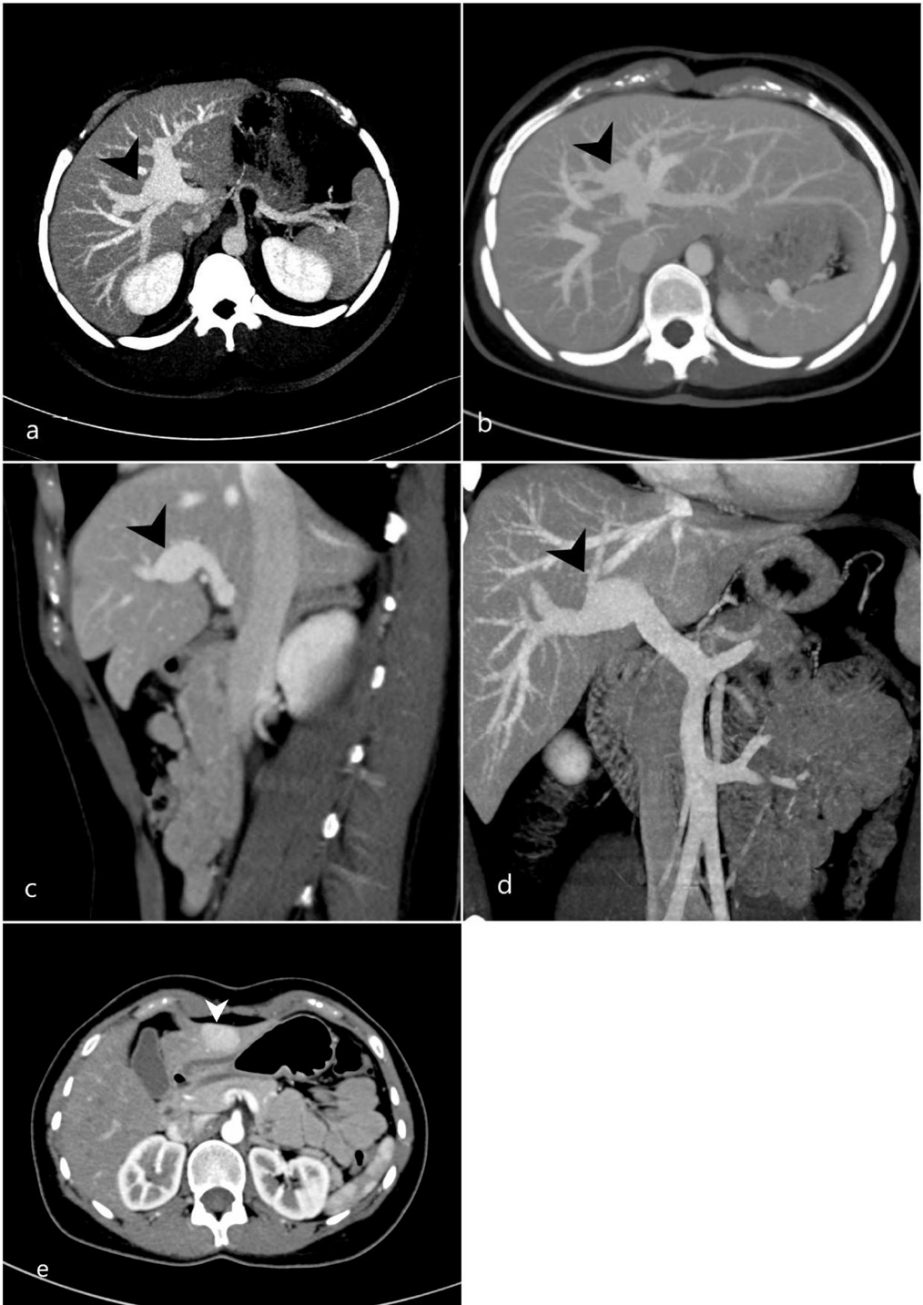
Axial (a, b), sagittal (c) and coronal (d) MIP images of an enhanced CT scan in portal venous phase showed a left intrahepatic portal vein aneurysm, measuring 1.6 cm in diameter (black arrowhead). (e) an incidental homogeneously enhancing lesion consistent with a focal nodular hyperplasia (white arrowhead). MIP, maximum intensity projection.
